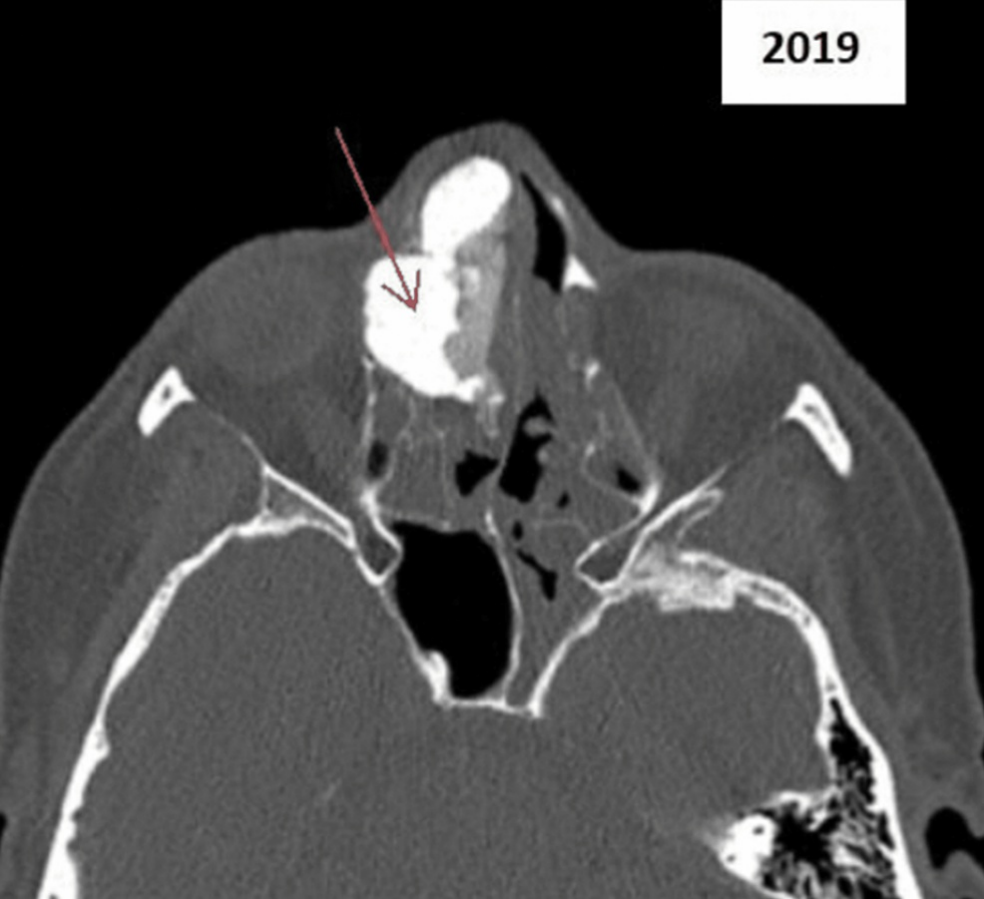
CT of sinuses in 2019 The red arrow is showing the osteoma.
